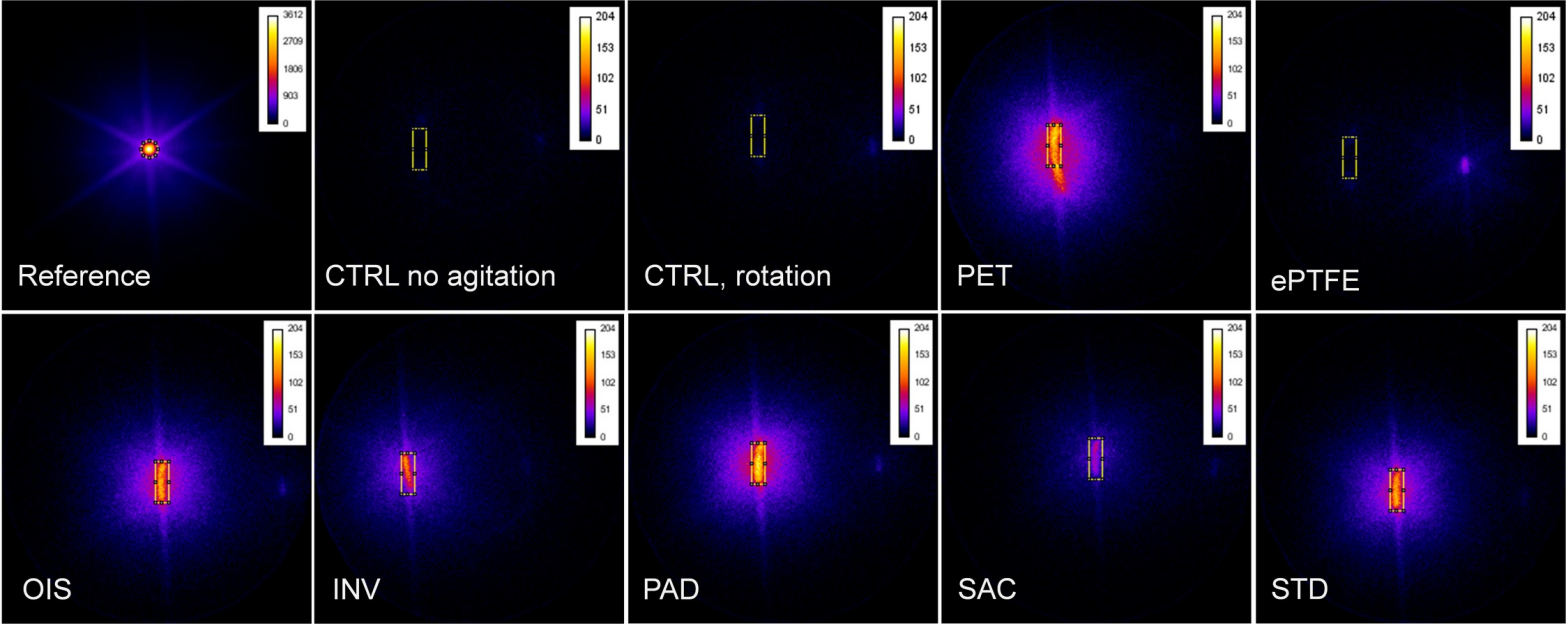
111In-oxine labelling of thrombocytes. Colored scintigraphies of the loops, with known amount of 111In-oxine labelled thrombocytes in whole blood, between different types of grafts succeeding 4 hours in a Chandler loop system. The yellow rectangle represents the region of interest for measurements of pixel values. The blue glow around the yellow/red shining BNC with adherent thrombocytes is an artifact. The color scale was set from black (no radioactivity) to white (high radioactivity) and a point source, 5.313 MBq, served as a reference. (CTRL: Control).
